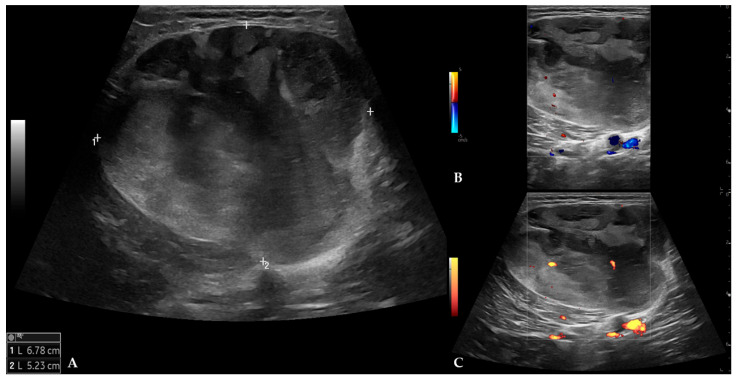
B-mode, color, and power Doppler images of MLS: (A) On B-mode, MLS presented as a heterogeneous mass with hypoechoic foci; (B) on color Doppler, MLS was poorly vascularized with a low arterial peak systolic velocity of 13 cm/s (not shown on image); (C) on power Doppler, MLS also demonstrated mild central vascularity.
